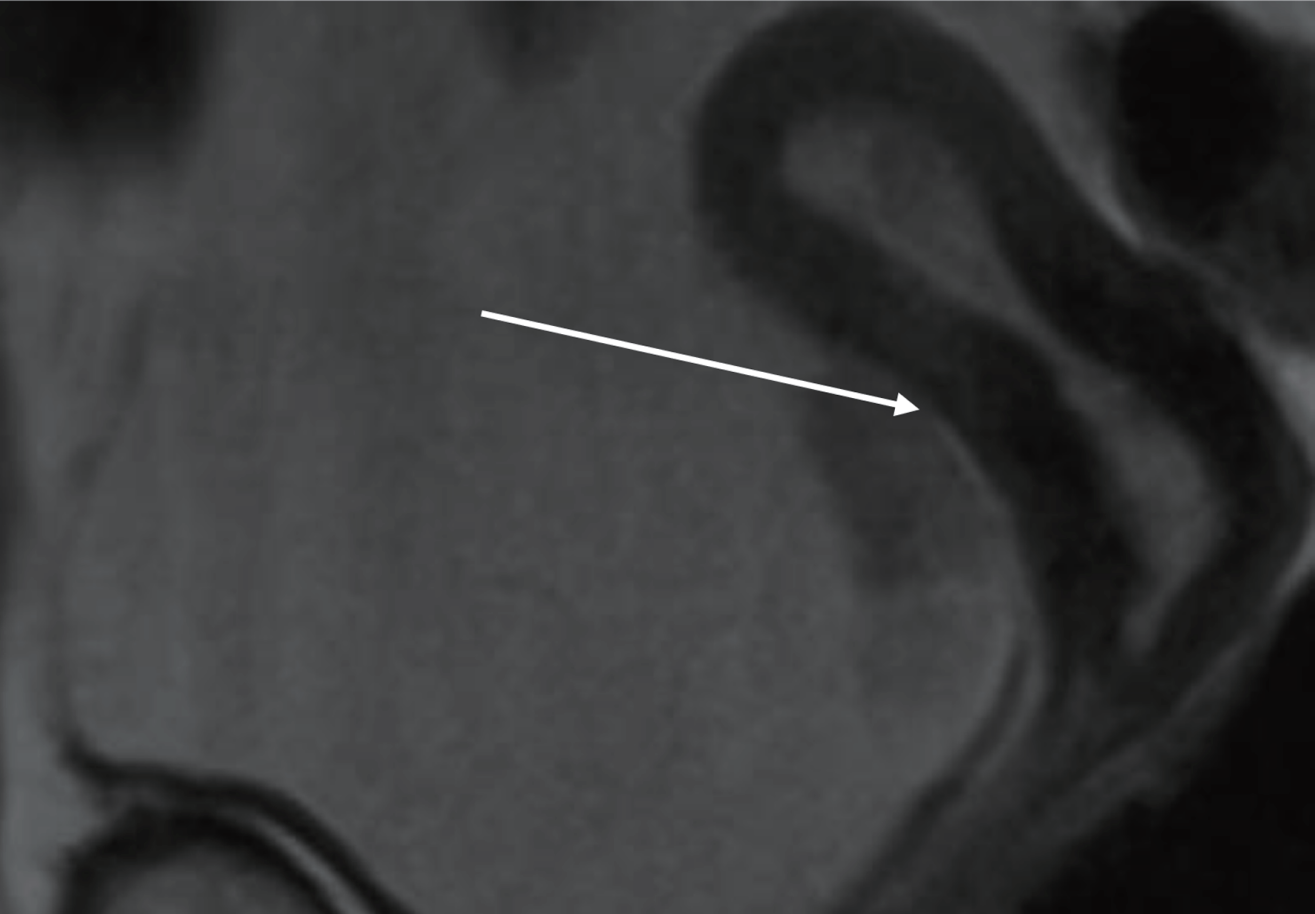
Lower abdominal magnetic resonance imaging (MRI) indicated possible infiltration of uterus (arrow).
